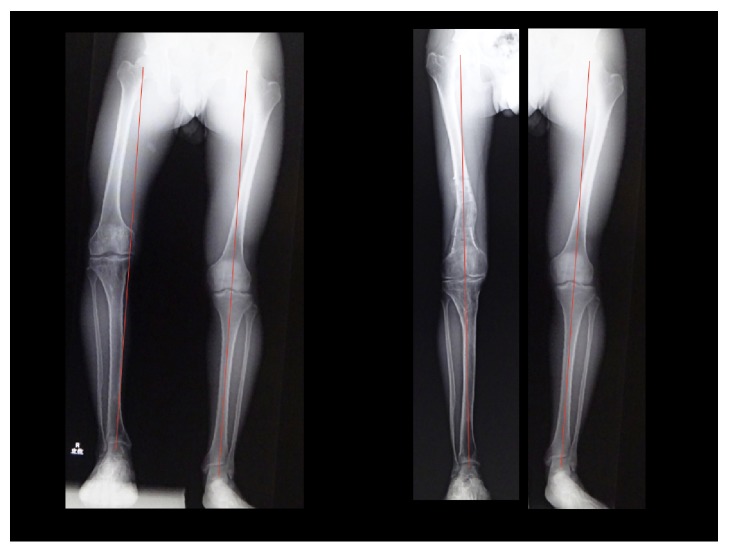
Correction of extra-articular deformity by using hexapod external fixator system. X-ray photos (a) before and (b) after reconstruction.
